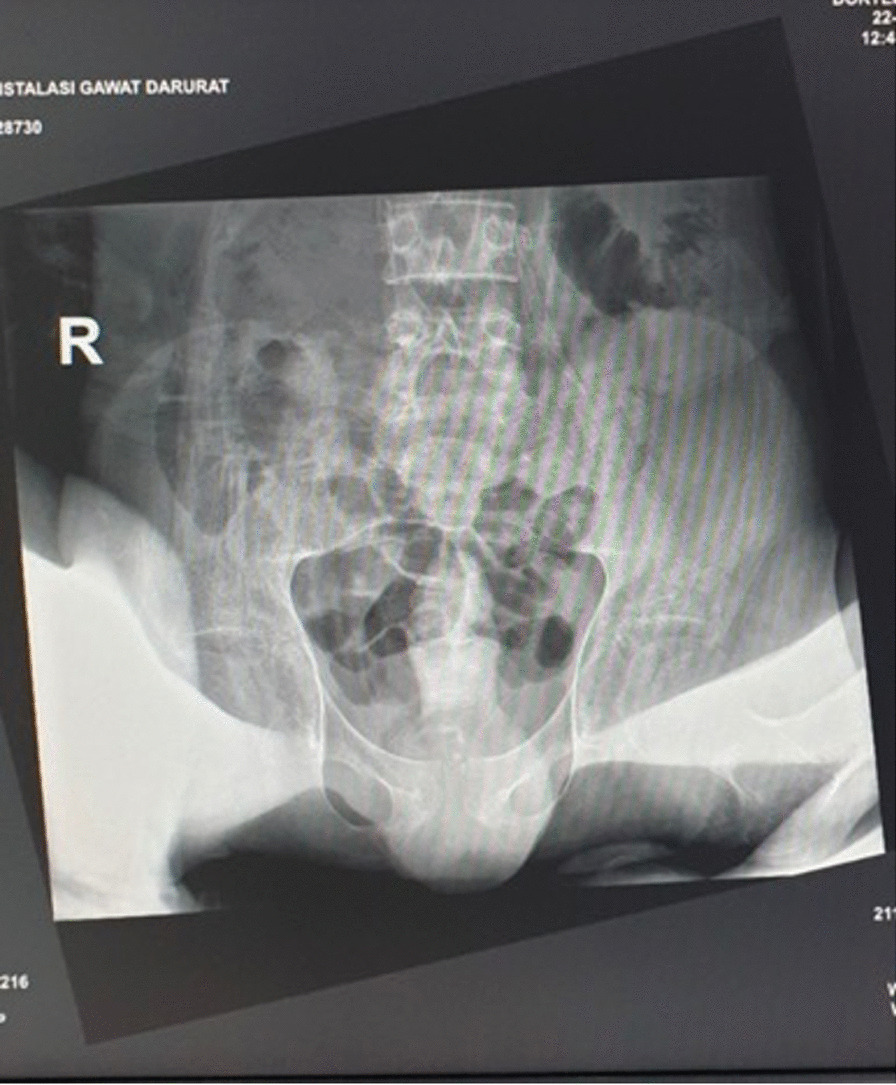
AP plain radiographs of pelvis, showing decreased density and porotic bone without fracture or bone destruction
